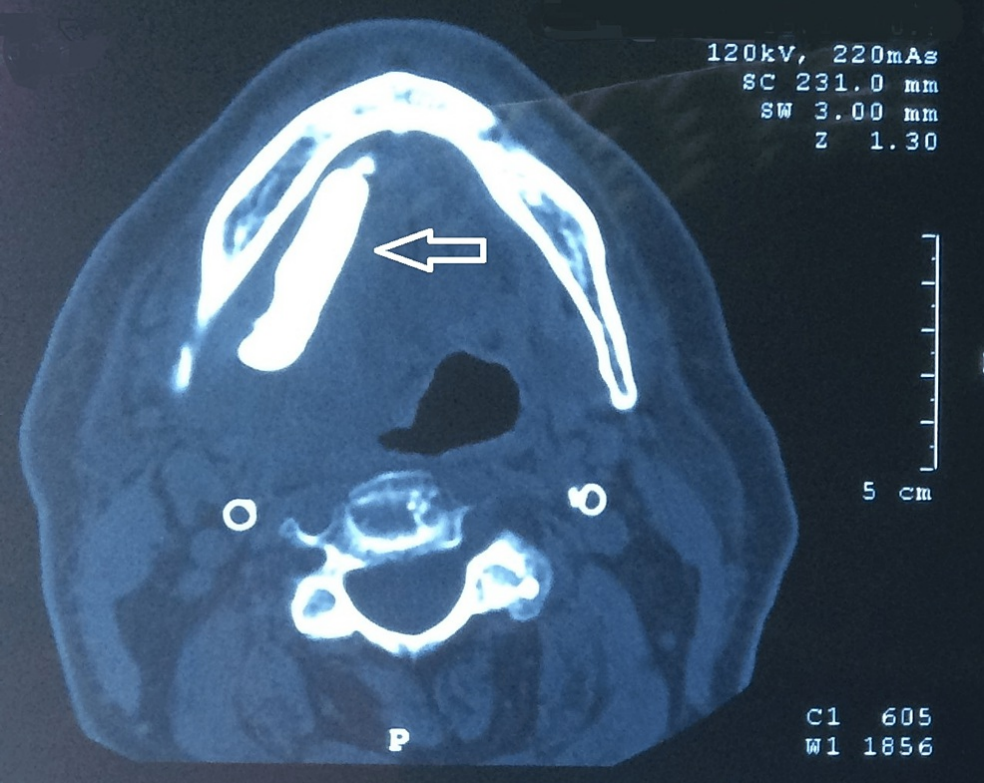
Computed tomography shows the presence of a giant stone in the duct of the right submandibular gland (white arrow).
